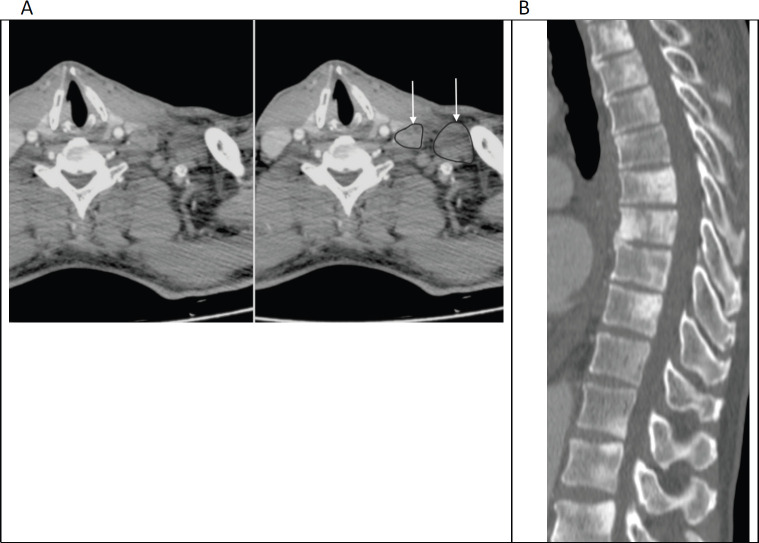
(a): Axial contrast enhanced CT of the lower neck/upper thorax demonstrating left supraclavicular adenopathy (unmarked on the left image and annotated on the right; with arrows pointing to outlined nodes) that was the initial presentation of prostate cancer in this 65-year-old man. (b): Sagittal CT (bone window) of the thoraco-lumbar spine in the same patient shows widespread sclerotic bone metastases, which were also present at the time of initial diagnosis.
